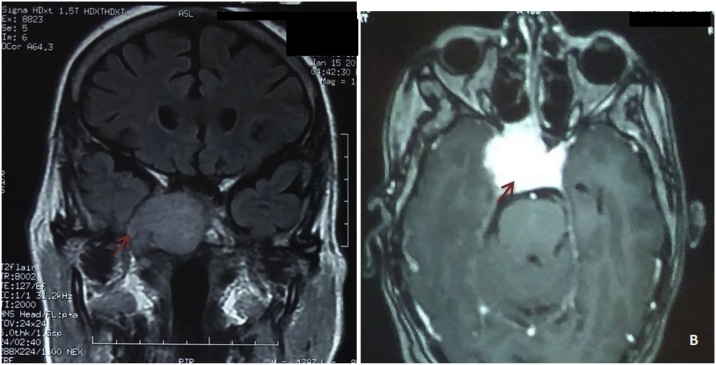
Frontal (A) and axial (B) T1-weighted MRI with gadolinium showing the tumor of the sella turcica.
